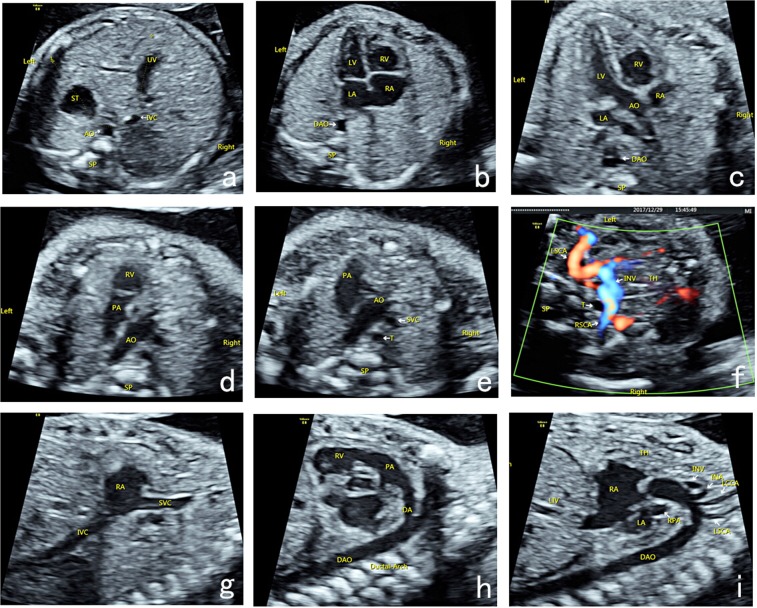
Nine-segment-sequential method for analysis of fetal heart ultrasound. Nine-segment-sequential method for analysis of fetal heart ultrasound included nine basic sections: transverse section of upper abdomen (a), four-chamber heart section (b), left ventricular outflow tract section (c), right ventricular outflow tract section (d), three-vascular-tracheal section (e), bilateral subclavian artery section (f), long axis section of superior and inferior vena cava (g), long axis section of ductus arteriosus (h), and long axis section of aortic arch (i). UV, umbilical vein; ST, stomach; IVC, inferior vena cava; AO, aortic; SP, spinal; DAO, descending aorta; LA, left atrium; RA, right atrium; LV, left ventricle; RV, right ventricle; PA, pulmonary artery; SVC, superior vena cava; T, trachea; TH, thymus; LSCA, left subclavian artery; RSCA, right subclavian artery; IVC, inferior vena cava; DA, ductus arteriosus; INV, innominate vein; INA, innominate artery; LCCA, left common carotid artery; LSCA, left subclavian artery.
